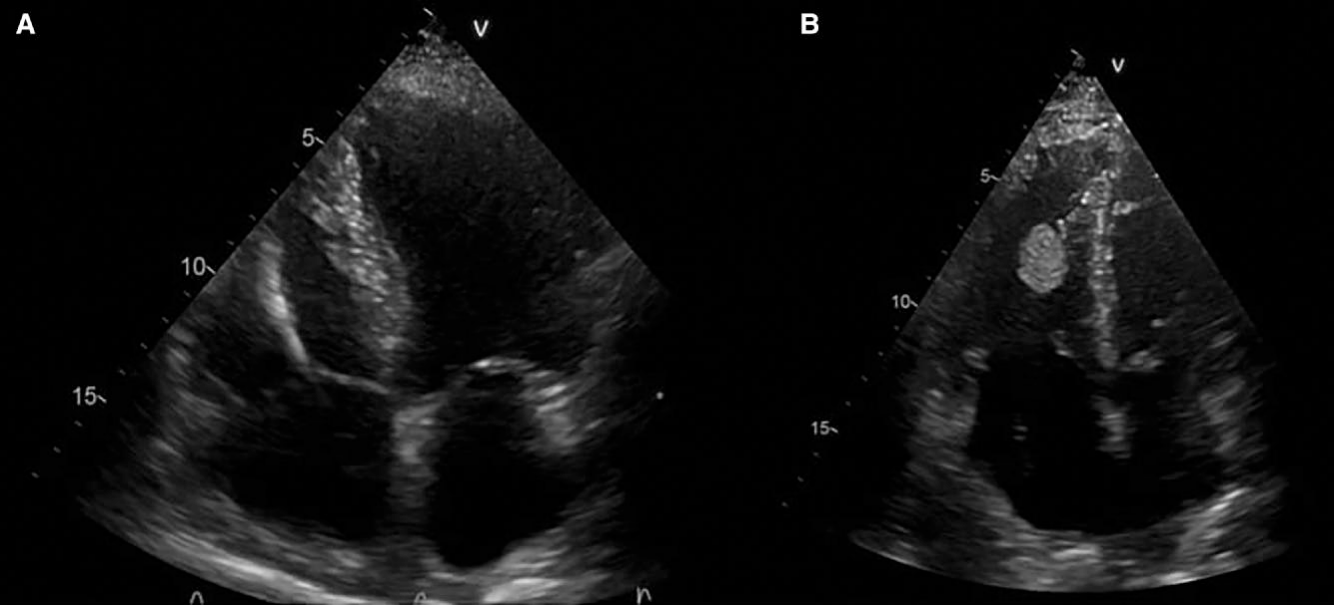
Transthoracic cardiac echocolordoppler a year before the diagnosis of intracardiac thrombus (*A*). Transthoracic cardiac echocolordoppler showing thrombus on pacing lead of right ventricle (*B*).
